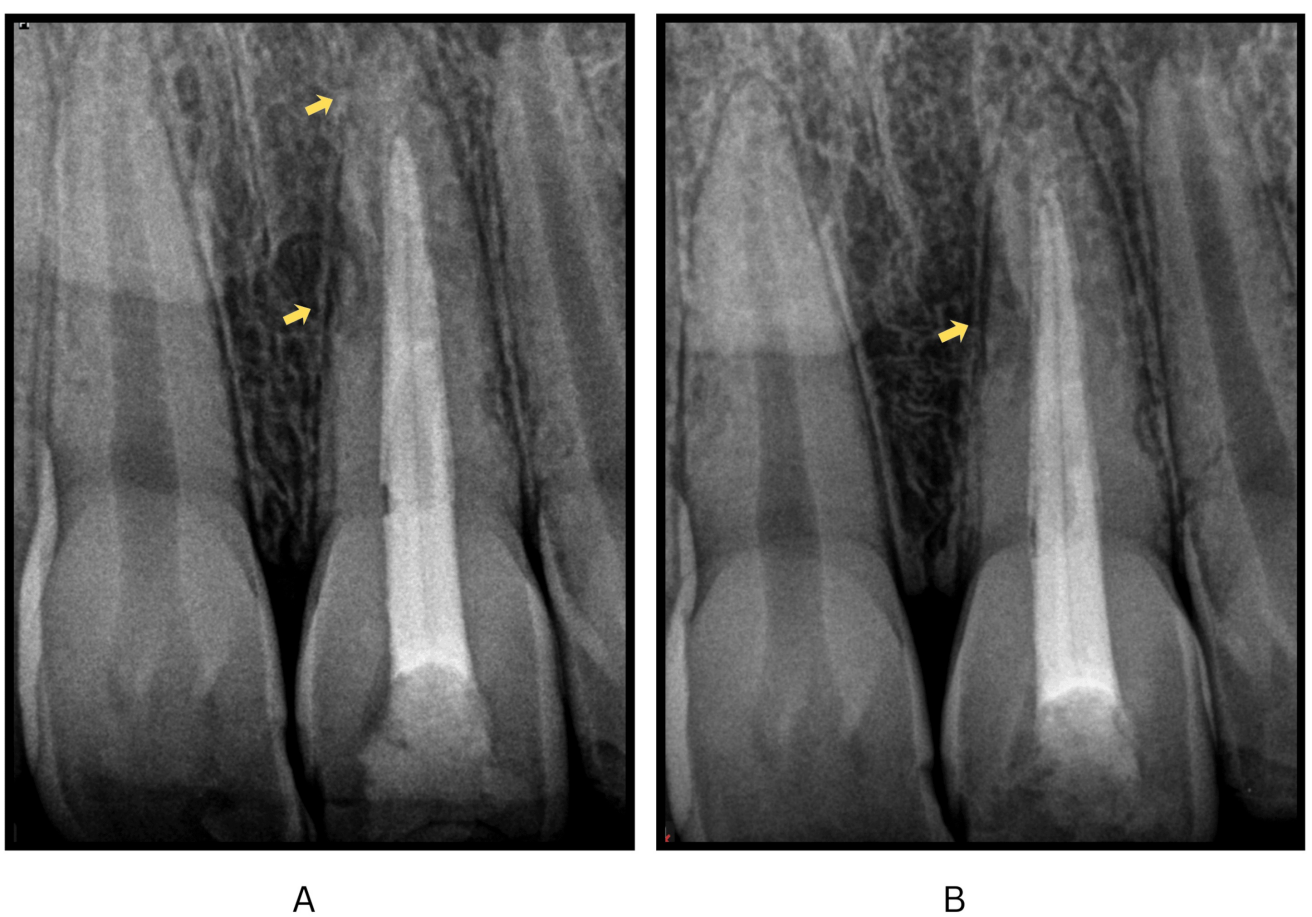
Long-term follow-up periapical radiographs demonstrating clinical stability of the replanted maxillary left central incisor. (A) Four-year follow-up radiograph showing an asymptomatic tooth #21. The arrows indicate the resolution of the lesion. (B) Five-year follow-up radiograph showing an asymptomatic tooth #21, with arrows indicating resolution of the lesion.
